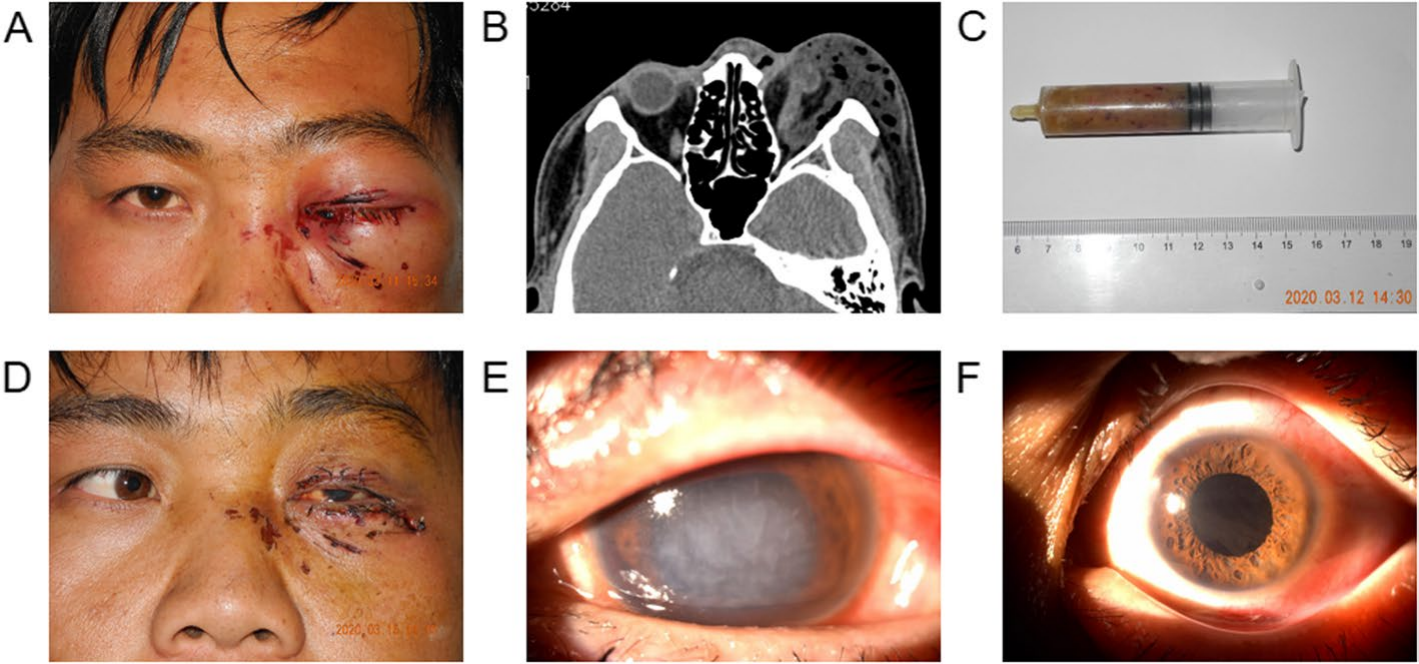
Case 1. A Appearance on presentation showing marked periorbital swelling with irregular wound, blood scab and proptosis. B Orbital CT showing significant axial proptosis and extensive inflammation with numerous bubble-liking hypodense masses within the left orbital and periorbital soft tissue. C The grease removed from the orbit. D Appearance on the third day after the initial surgery showing reduced periorbital swelling and proptosis with a remarkable restriction of abduction of the left eye. E The view of the anterior segment of the left eye on the 3rd day after the initial surgery showing corneal edema, dilated pupil, lens opacity. F The view of the anterior segment of the left eye on the 7th day after the second surgery
